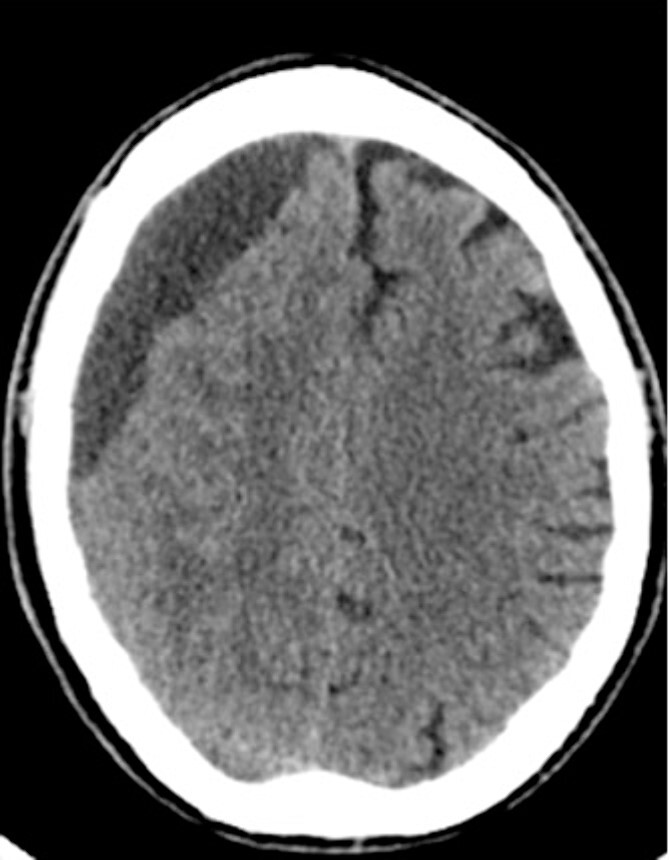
Preoperative CT imaging from Case 2.
